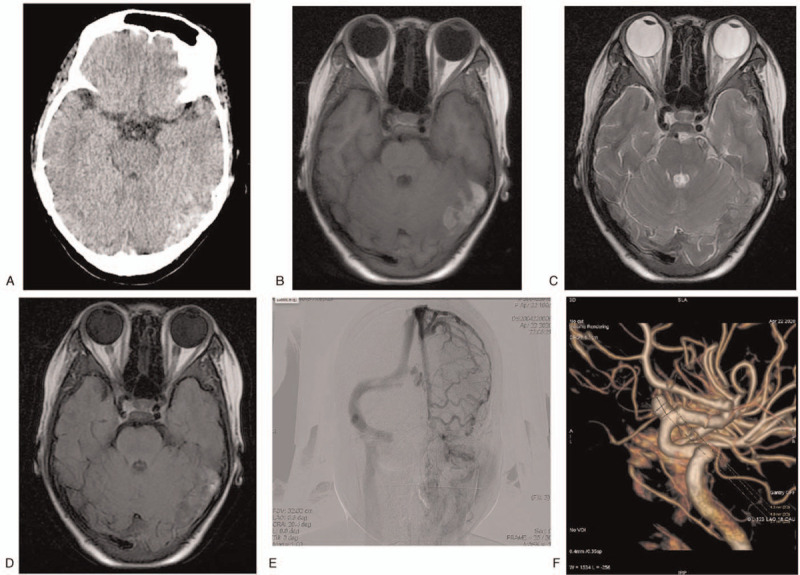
(A). Brain CT showed little hemorrhage in the left occipital lobe. (B). Brain MRI(T1WI) showed high signal lesion located in the left lateral transverse sinus and sigmoid sinus area of the left occipital region. (C). Brain MRI (T2WI) showed low signal lesion. (D). Brain MRI (FLAIR) showed mixed signal lesion. (E). DSA showed left transverse sinus and sigmoid sinus occlusion, and filling defect of right transverse sinus. (F). DSA showed two aneurysms located in the C6 segment of the right ICA with the size of 4.9^∗^5.7 mm and 2.1^∗^1.3 mm.
